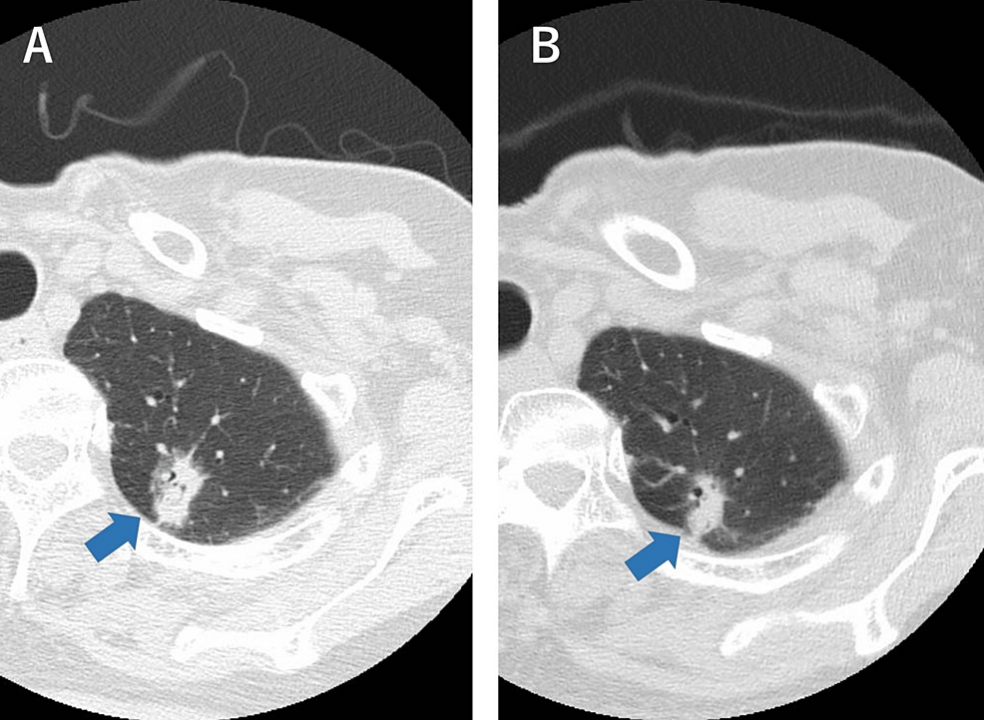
Chest computed tomography Computed tomography obtained (A) before and (B) three months after stereotactic ablative radiotherapy for lung cancer in the left upper lobe. Blue arrows indicate the lesion.
